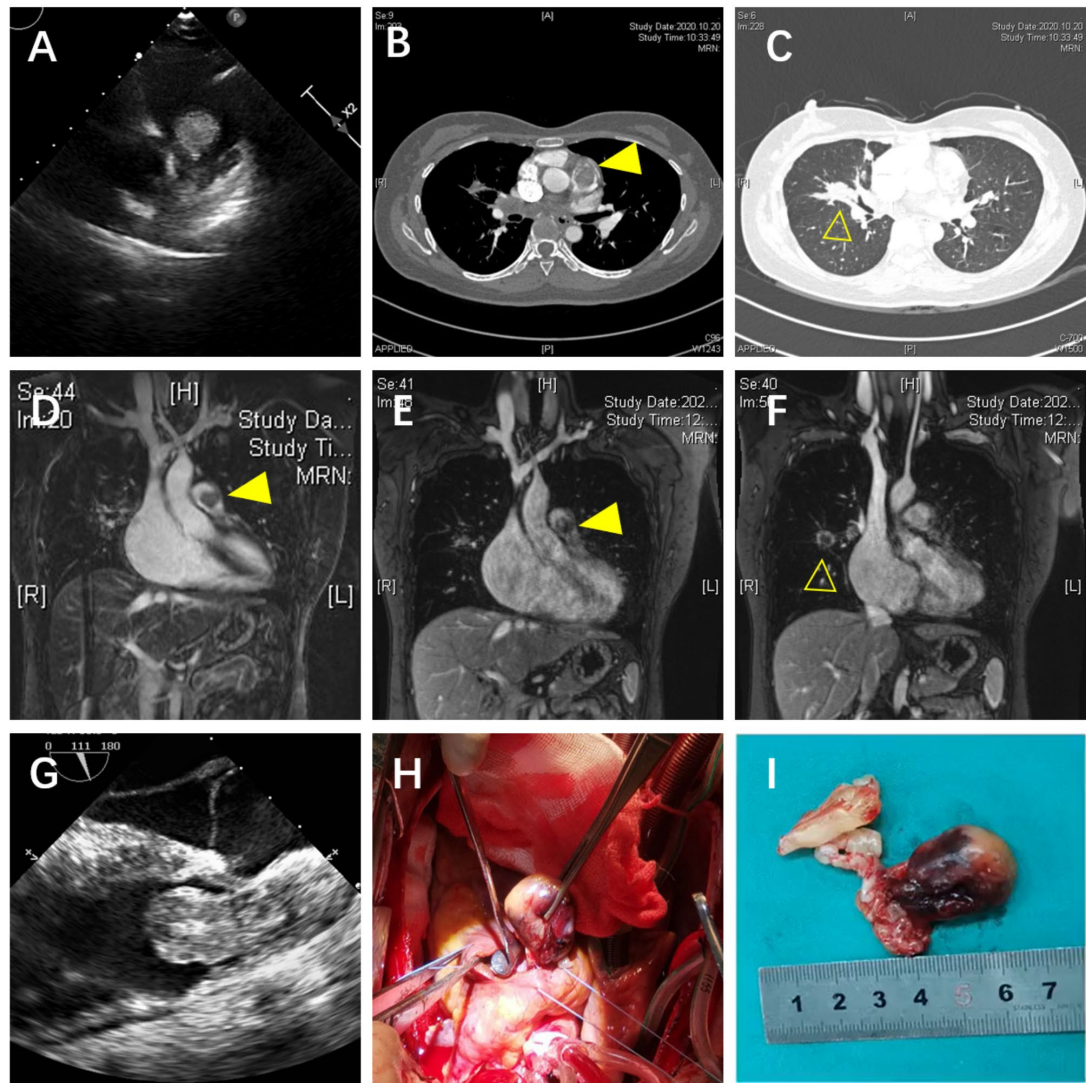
Transthoracic echocardiography (TTE) showing the space-occupying lesions of the right ventricular outflow tract (RVOT) (A). Computed tomography pulmonary angiogram (CTPA) revealed that RVOT obstruction was caused by the mass (B) and the presence of lesions in the right lung (C). The tumor was poorly demarcated with the right ventricular wall and enhanced on MR images of the pulmonary artery (D-E). Inhomogeneous enhancement nodules in the middle lobe of the right lung (F). Transesophageal echocardiography (TEE) showing the multiple pedicled masses with hyperechoic capsule (G). Intraoperative and gross appearance of the tumors (H, I)
